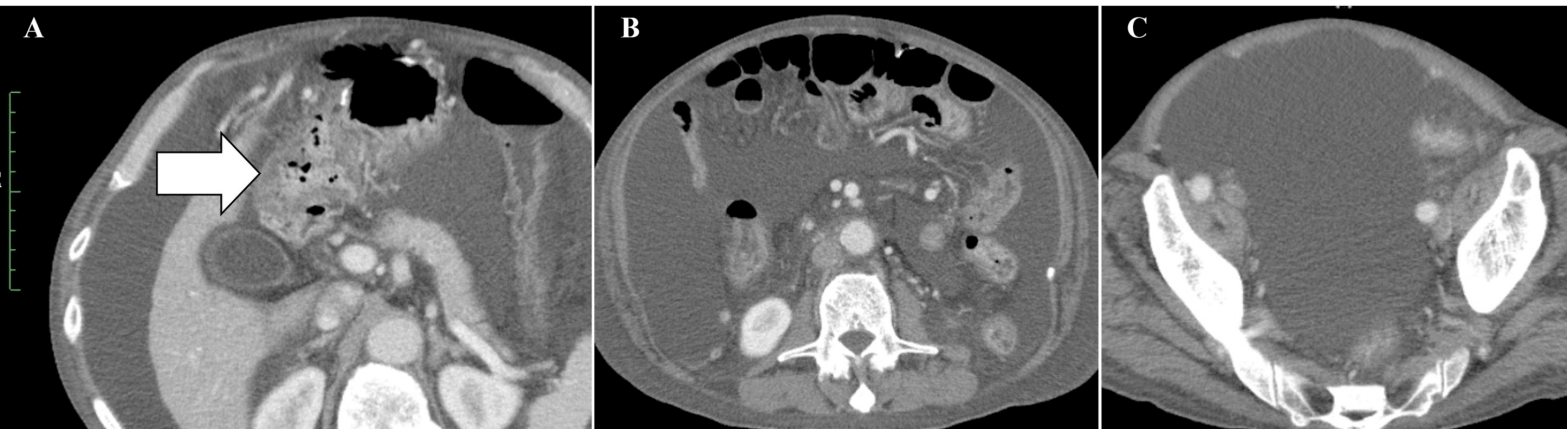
Abdominal CT taken before ACT. (A) A grossly enlarged omentum was observed surrounding the duodenum (arrow). (B, C) A considerable amount of ascites resulting from the dissemination of duodenal cancer was detected. ACT, adoptive cell therapy.
